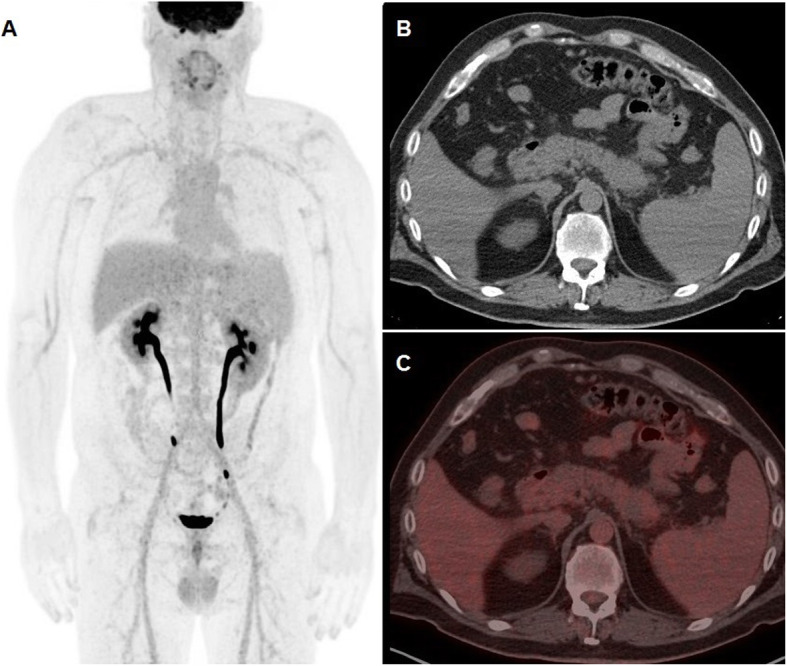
Whole body PET/CT (A), CT (B) and fused PET/CT (C) slices showed complete regression of the hypermetabolic lesions previously described in the left arm and pancreas
